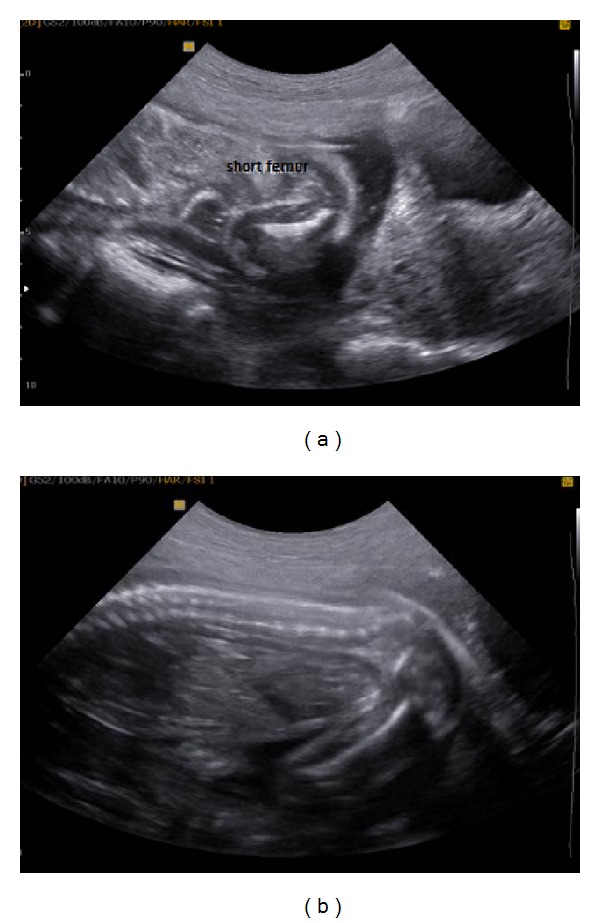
Ultrasound images of both the right and left femurs at 20 wg. Left femur: 19.7 mm and the right femur: 31.6 mm were measured.
